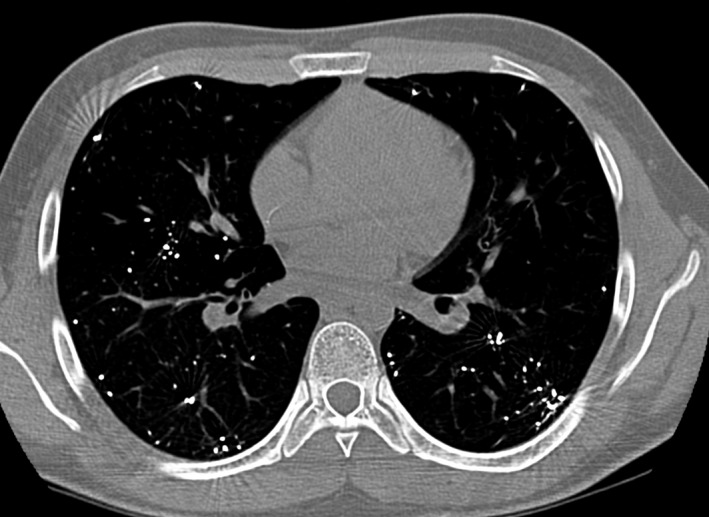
Coronal thoracic computed tomography shows the presence of multiple images of radiopaque material in both lung fields
